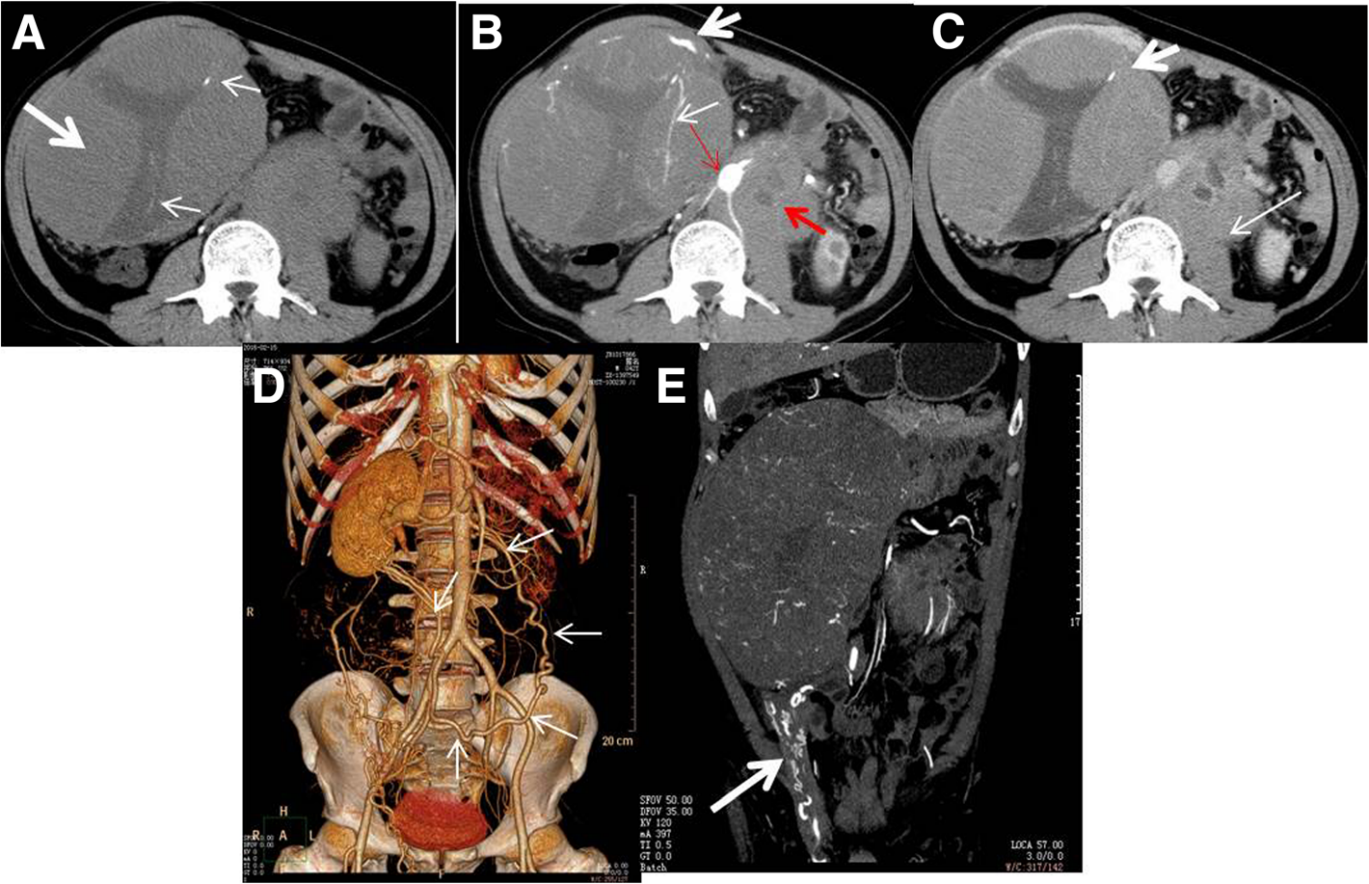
A 48-y-old male patient. a A CT plain scan showed that the tumor was mainly solid (thick arrow), with a CT value of 40 HU. A T-shaped low-density area was visible in the central area of the solid part of the tumor, and the CT value was 28 HU. Dots or striped calcifications were visible in the T-shaped low-density area (short thin arrow). b In the arterial phase, a thickened angiogram (arrow) was visible in the lateral margin of the tumor and distributed throughout the solid part of the tumor. The area containing the striped calcifications was slightly enhanced (thin arrow). Para-aortic lymph nodes were enlarged, and blood vessels were embedded. Vascular morphology was complete, and significant enhancement was found in the arterial phase (red thin arrow). Metastatic lymph nodes showed low density enhancement (red thick arrow), indicative of the floating ice sign. c The solid part of the tumor showed progressive enhancement, and no enhancement was seen in the low-density area. A tortuous artery was visible at the margin of the tumor (thick arrow). Enlarged lymph nodes showed progressive enhancement, and no enhancement was seen in necrotic areas (thin arrow). d The coronal reconstruction displayed a right varicocele (arrow), but the left varicocele was not visible. e VR images clearly displayed the origin and distribution of the blood vessels and the supply of the tumor (arrow)
